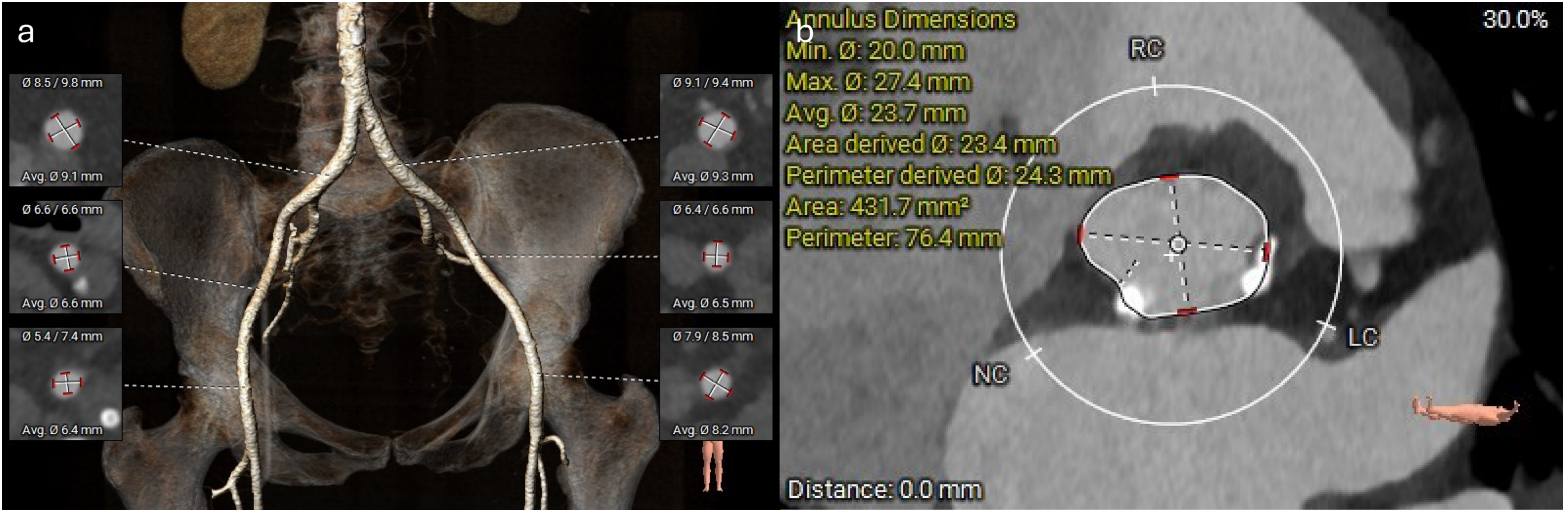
CT reconstructions of the femoral vessels and aortic annulus. (a) 3D reconstruction of the femoral arterial access. (b) Measurements of the aortic annulus.
